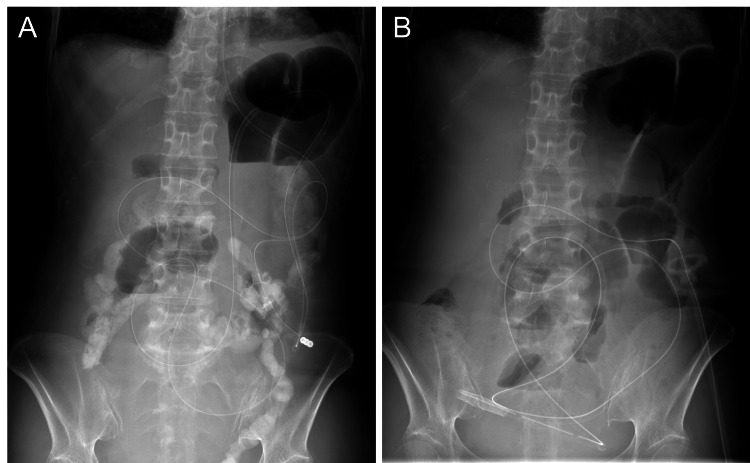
Abdominal radiography after nasojejunal tube placement and PEG-J (A) Abdominal radiograph (standing position) after nasojejunal tube placement showing partial reduction of air-fluid levels. (B) Abdominal radiograph (standing position) after replacement with PEG-J PEG-J: percutaneous endoscopic transgastric jejunostomy
